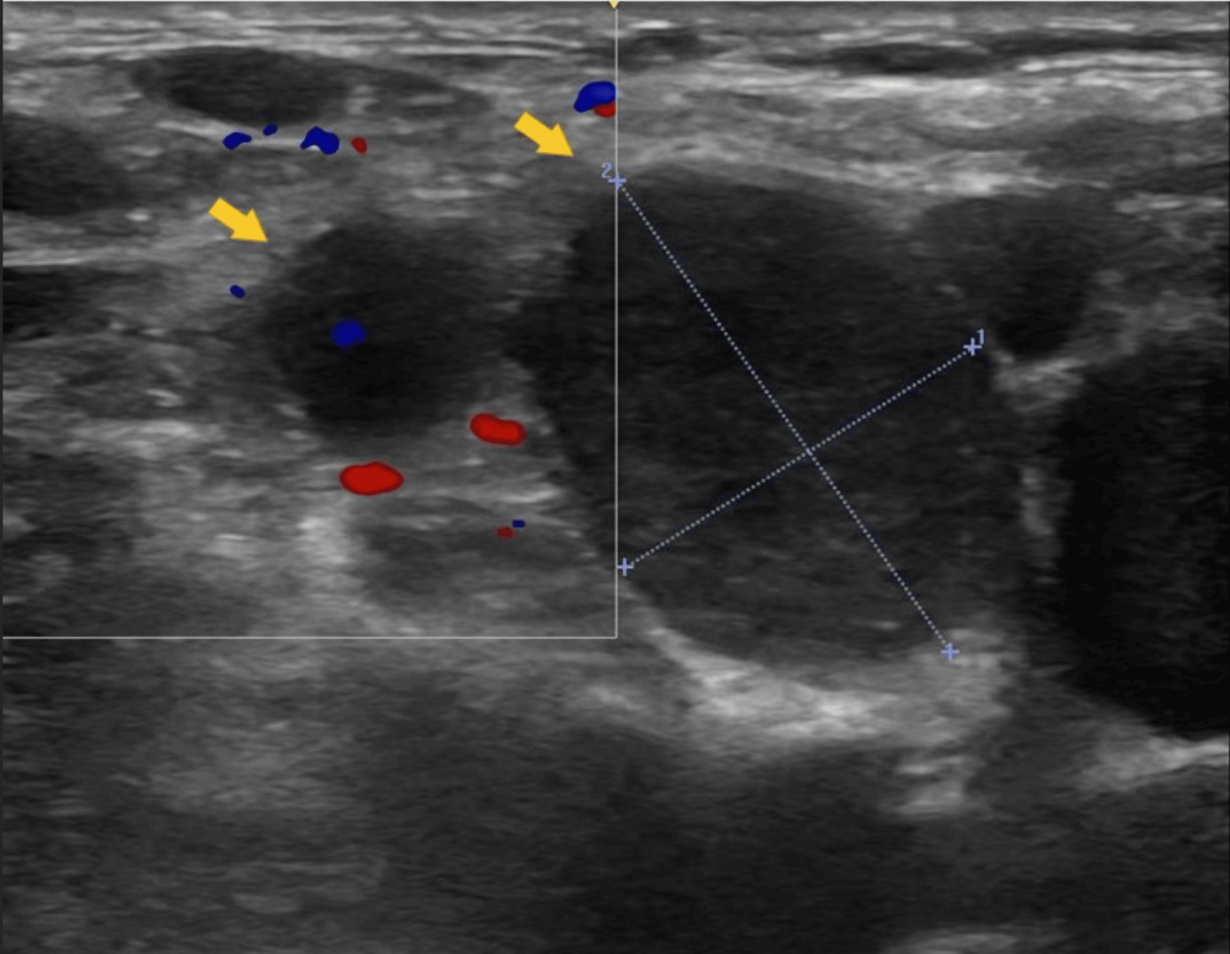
Bedside neck ultrasound revealed multiple enlarged, heterogeneous, rounded to irregularly shaped lymph nodes (yellow arrows) lacking visible hila, suggestive of a malignant etiology.
